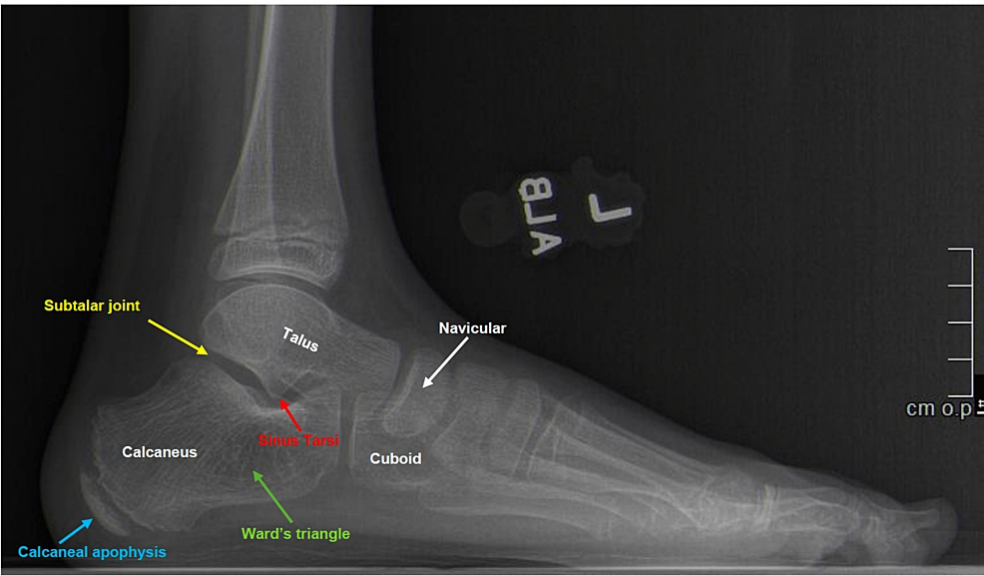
Lateral weightbearing radiograph of the foot. Major anatomical landmarks of the hindfoot are labeled including the calcaneus and its surrounding structures. The calcaneus articulates with the bone directly superior to it, the talus, via the subtalar (or talocalcaneal) joint. The sinus tarsi is a “canal” located at the lateral ankle that is formed between the talus and calcaneus. The calcaneal apophysis is part of the growing portion of the calcaneus that acts as a site of attachment for the Achilles tendon. This is one of the most common sites of heel pain in children. Ward’s triangle is a hypodense region within the calcaneus that is more susceptible to fracture and can be the site of calcaneal cyst formation.
